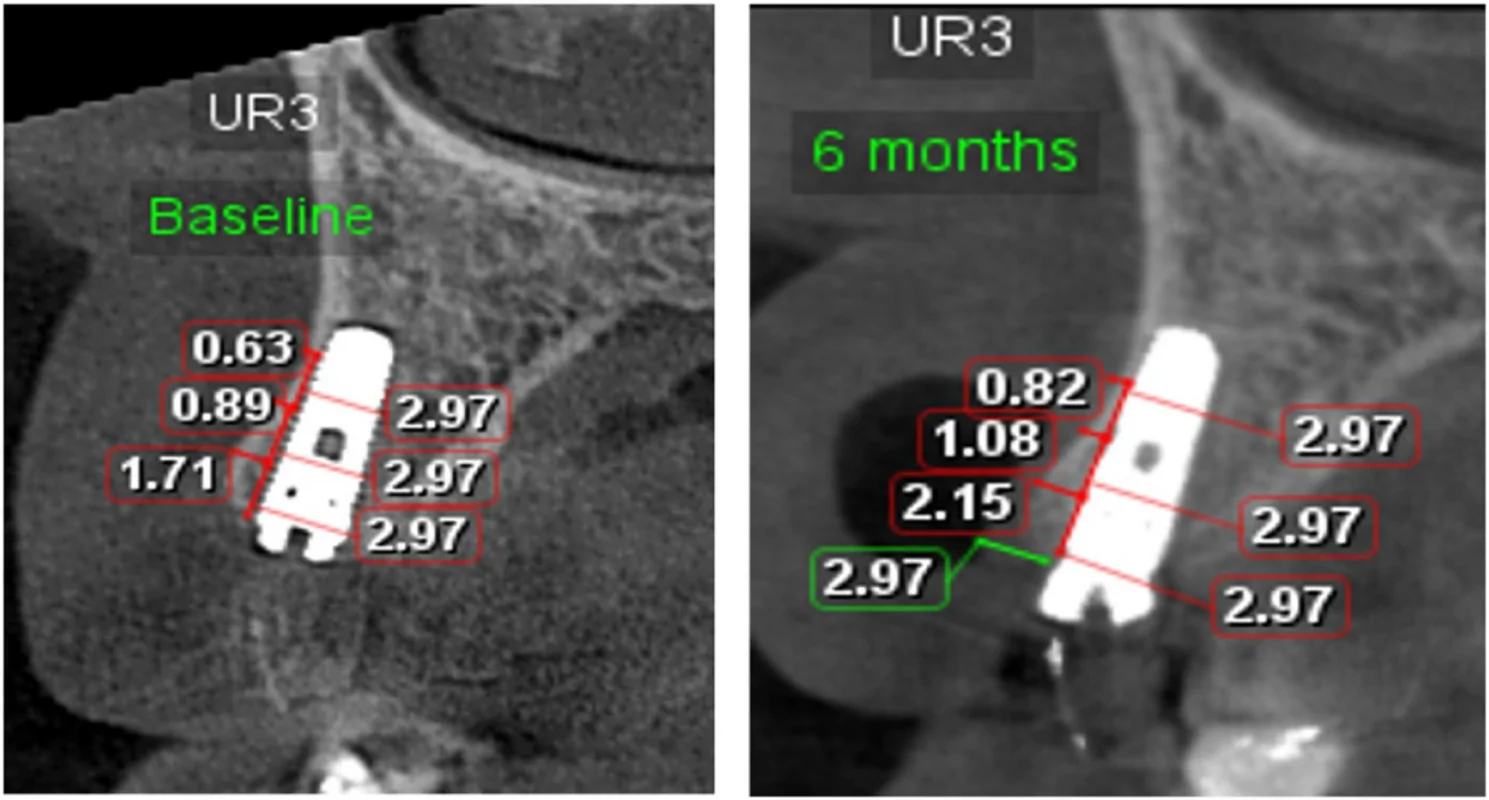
CBCT evaluation of labial bone thickness at baseline and 6 months following Fascia lata membrane
